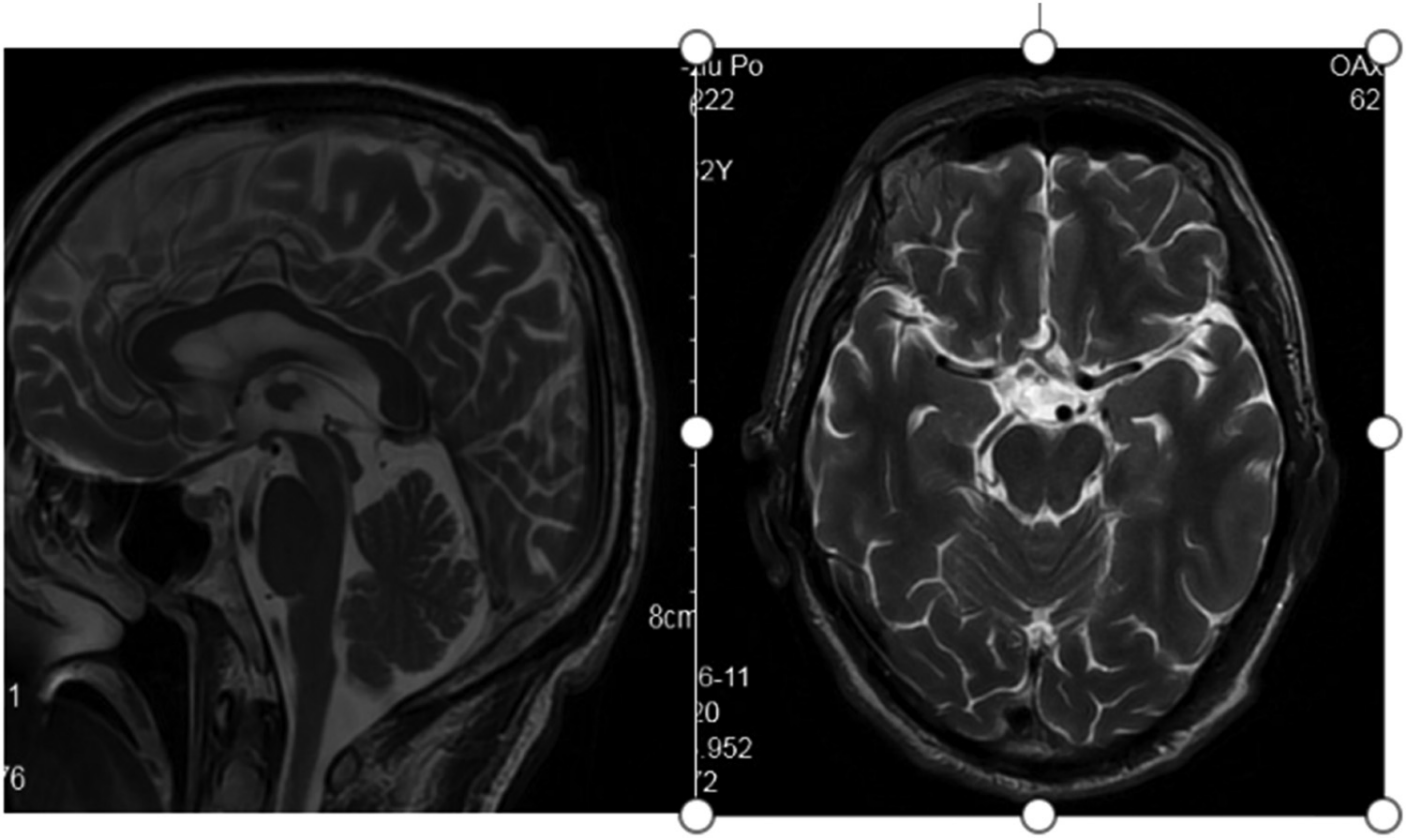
Flat pons, not full and mild cerebellar atrophy in T2-weighted MRI.
